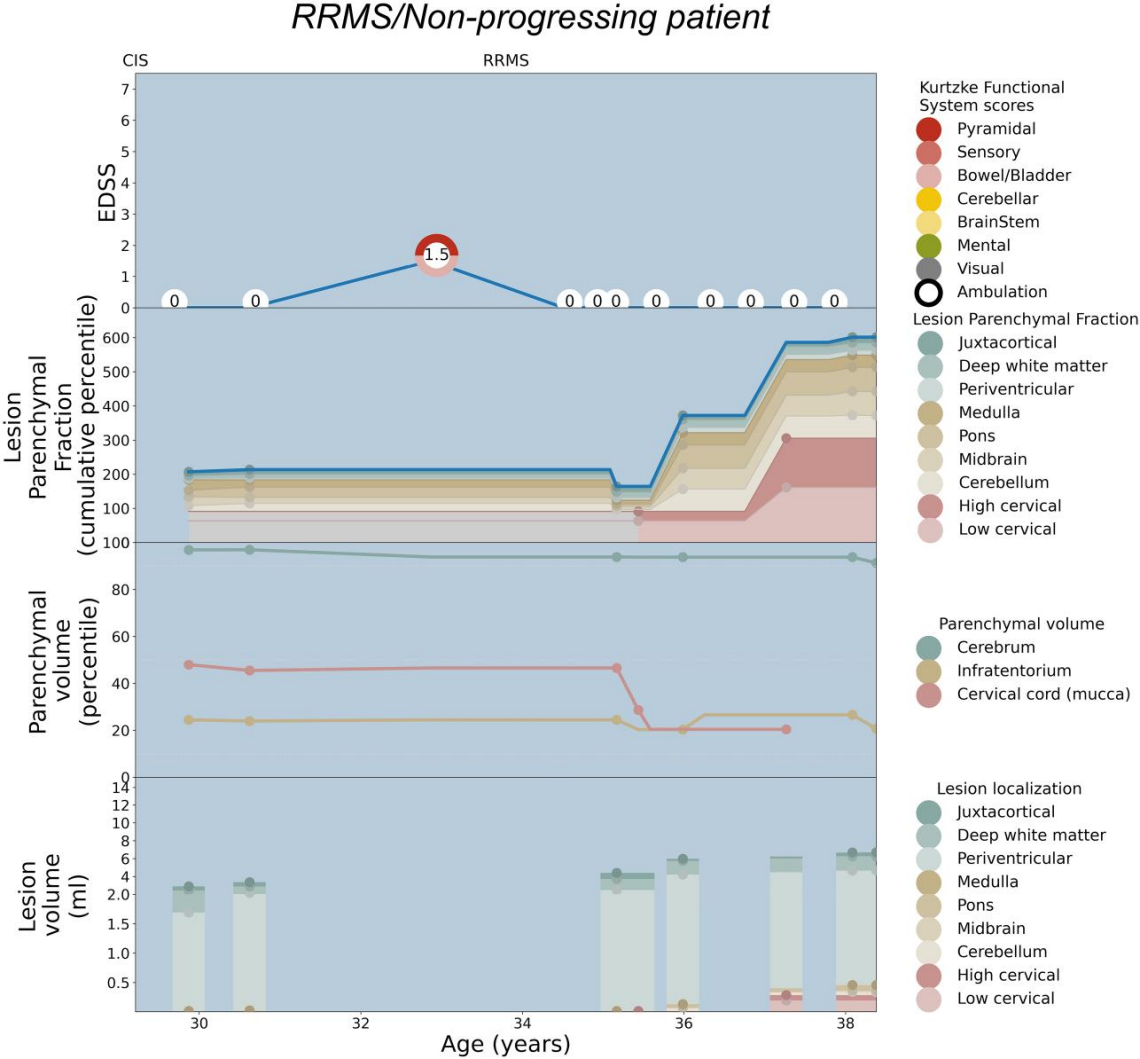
Individual patient outlier, demonstrating a more favourable clinical course compared to the predicted disability trajectory based on LPF. See the legend of Fig. 3 for a general explanation of the figure panels. This patient has a disability trajectory that does not track with the cumulative LPF measure of disease burden, with a better clinical course than would be predicted by the LPF model. She demonstrates minimal to no overt disability throughout the follow-up time over 9 years. While the initial LPF percentile is commensurately low (noting the absence of cord data during this period depicted in grey) at the age of 36, the LPF starts to increase, initially driven by infratentorial lesions, and at the age of 37 also by emerging spinal cord lesion load and rising cord LPF. This did not translate into increased disability, with the EDSS remaining 0 at age 38. While this could be attributed to MRI measurement error—where the model causes small changes in cord lesion or parenchymal volume to have an outside effect on cumulative LPF—it is possible that the high brain parenchymal volume (as seen in the second panel from the bottom) is indicative of a higher level of reserve sufficient to compensate for the lesion burden.
